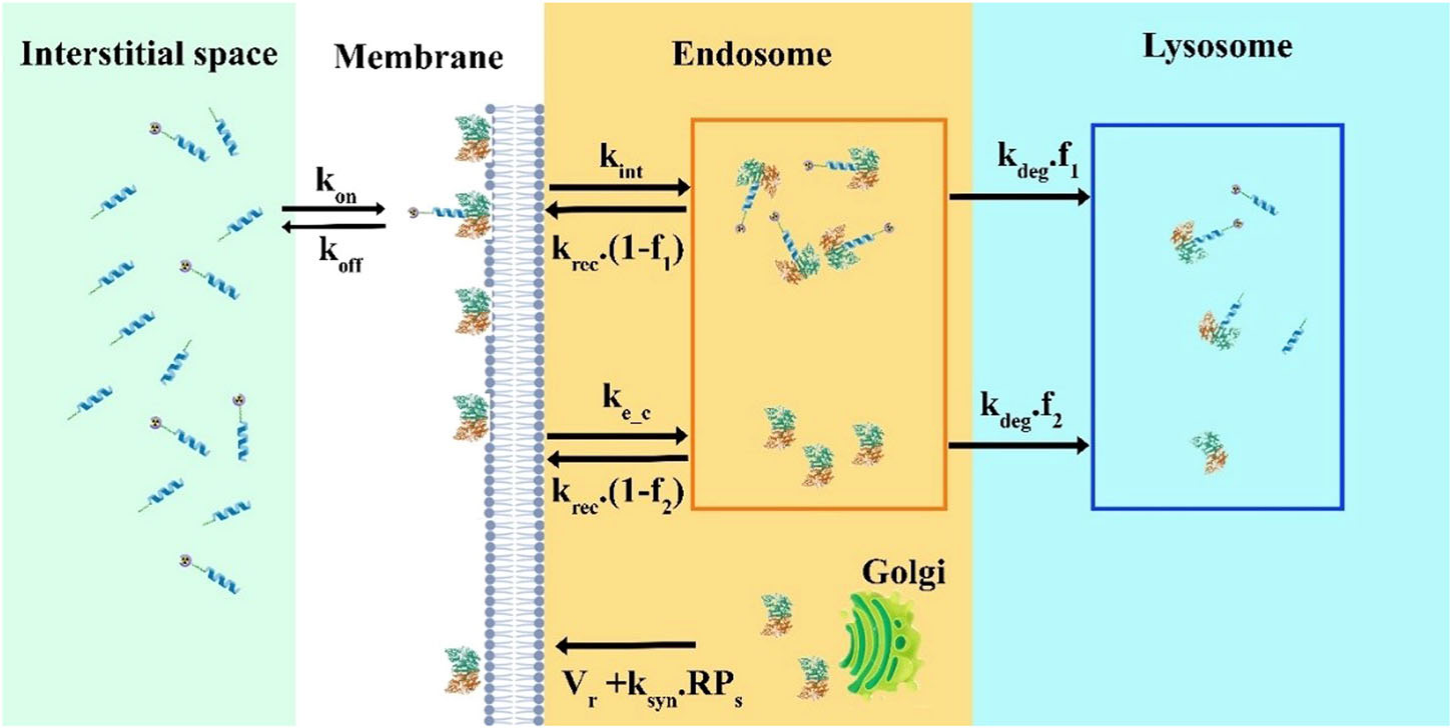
Model structure. PSMA ligands in interstitial space associate/disassociate reversibly to free PSMA receptors with the rate of k_off_ and k_on_. Ligand receptors and free PSMAs are endocytosed with the rate constant of k_int_ and k_e,c_, respectively. During internalization, a fraction of PSMA is recycled back to the plasma membrane at a constant rate (k_rec_). The remaining receptors (f_1_, f_2_) are degraded in the maturing endosome and eventually in the lysosome (k_deg_). Total PSMA receptor biosynthesis combines constitutive V_r_ and ligand-induced synthesis k_syn_.
